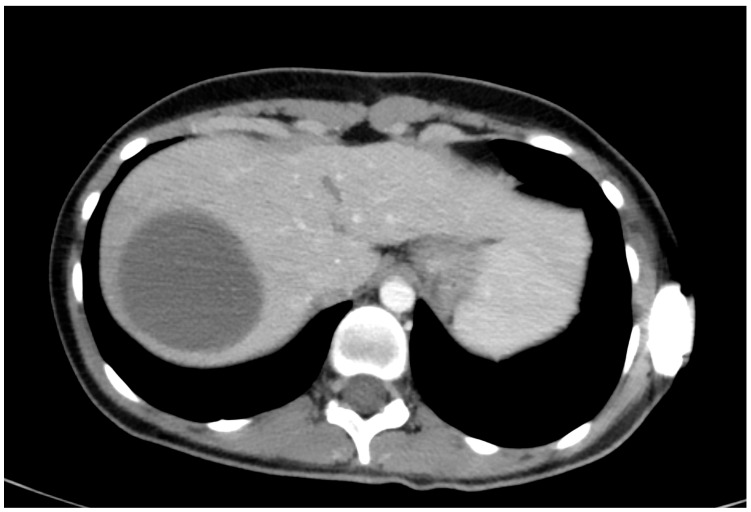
CT scan in patient with intraparenchymal haematoma and conservative treatment.
